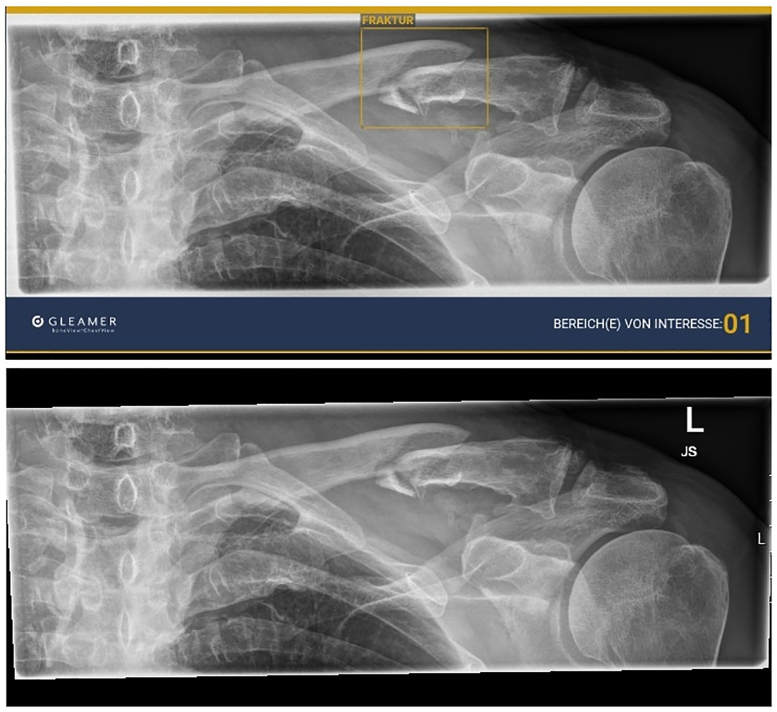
Native AP radiograph of a clavicle fracture and detection by the BoneView AI system. AP = anteroposterior.
